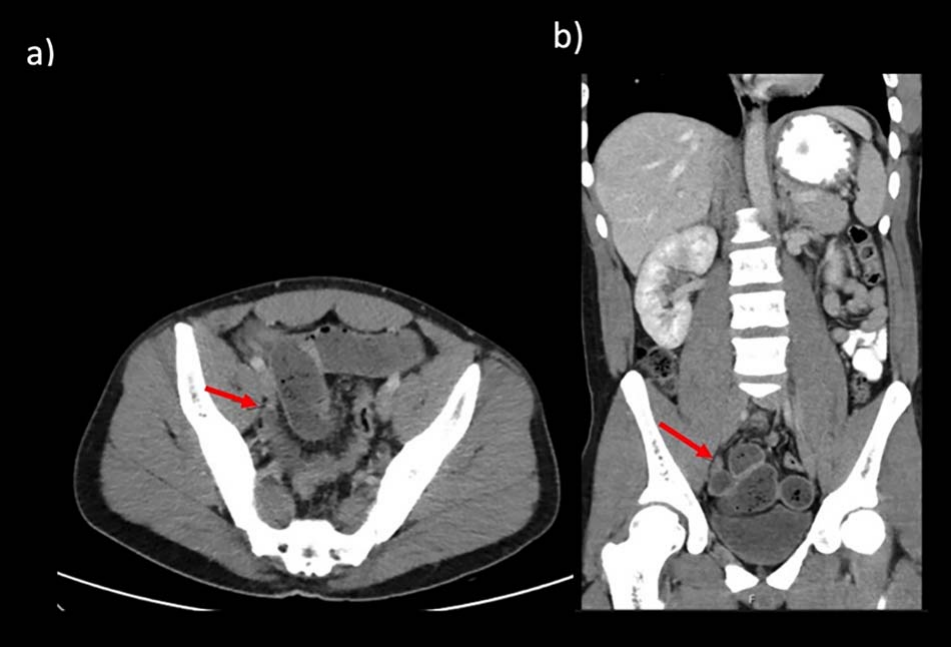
CT abdomen findings from Cases 1 and 2 showing distal SBO with the transition point in the pelvis. (a) Case 1 CT axial view. (b) Case 1 CT coronal view.
